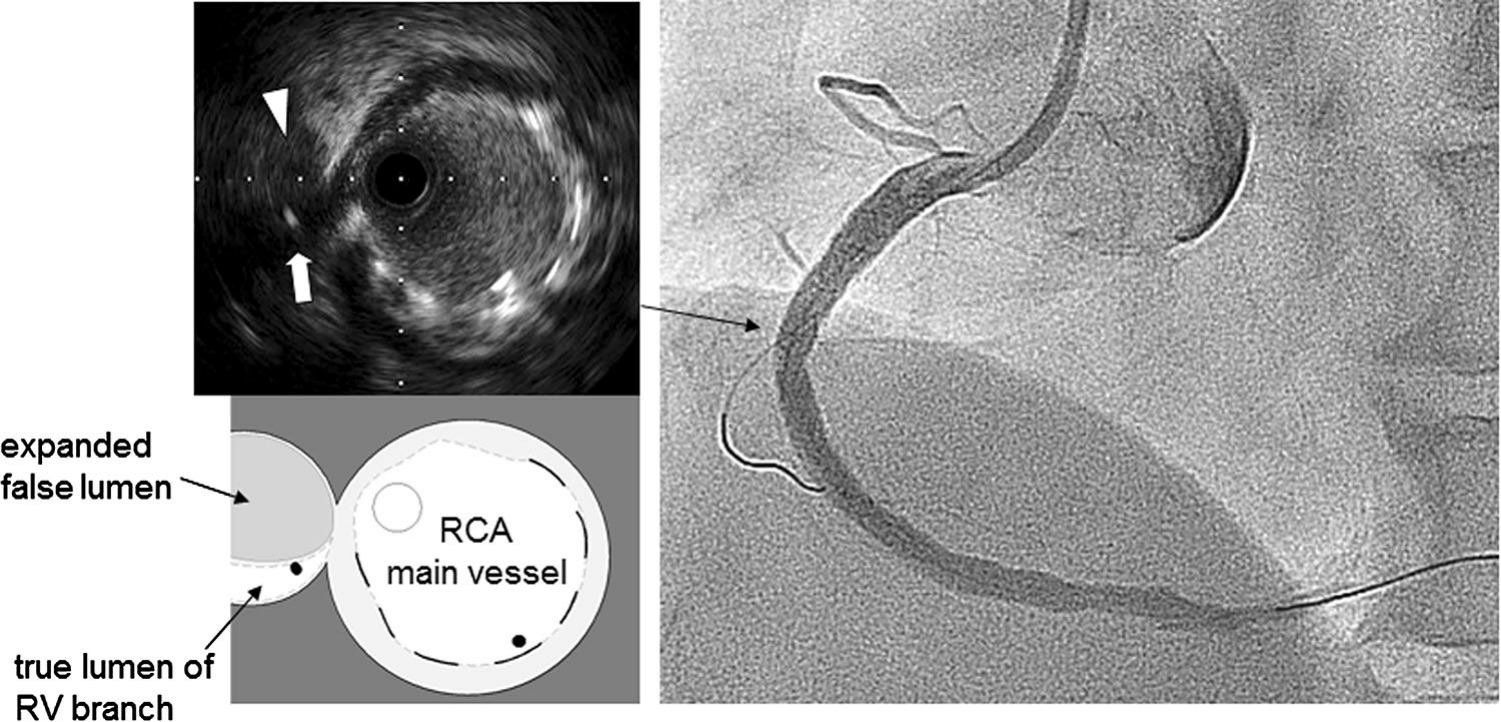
Angiogram after stenting showed that the right ventricular (RV) branch remained completely occluded. Intravascular ultrasound image from the right coronary artery main vessel at the RV branch bifurcation revealed that the RV branch was detected at 9 o’clock position. The true lumen of the RV branch (*white arrow*) was collapsed by an expanded false lumen that was presented as a high echogenic lumen (*white arrowhead*)
